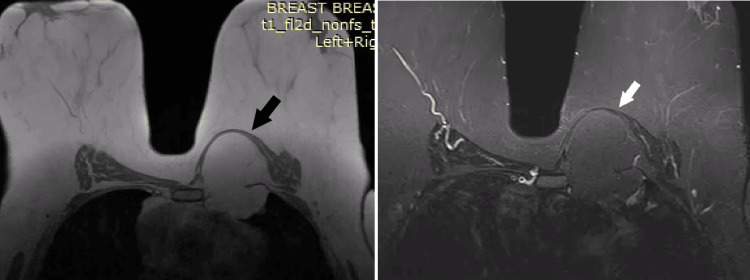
Magnetic resonance imaging of a dumbbell-shaped combined extra- and intrathoracic lipoma. (A) Axial T1-weighted 2D gradient-echo (FLASH), non-fat-suppressed image. (B) Axial T1-weighted fat-suppressed image. White and black arrows indicate the lesion.
